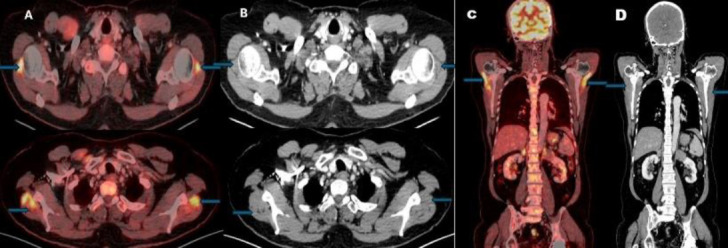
Fused PET/CT (A) and CT only (B) show increased FDG uptake at bilateral shoulder periarticular muscles in lateral aspect, and along supraspinatus/infraspinatus and teres muscles, without a focal mass or structural abnormality on CT (blue arrows). Coronal fused PET/CT images show diffuse increased FDG uptake along the scapular muscles (C) and corresponding CT images (D) (blue arrows) demonstrate no infrascapular soft tissue mass or typical alternating fat fibrous streaks helping exclude elastofibroma dorsi as the cause of FDG uptake
